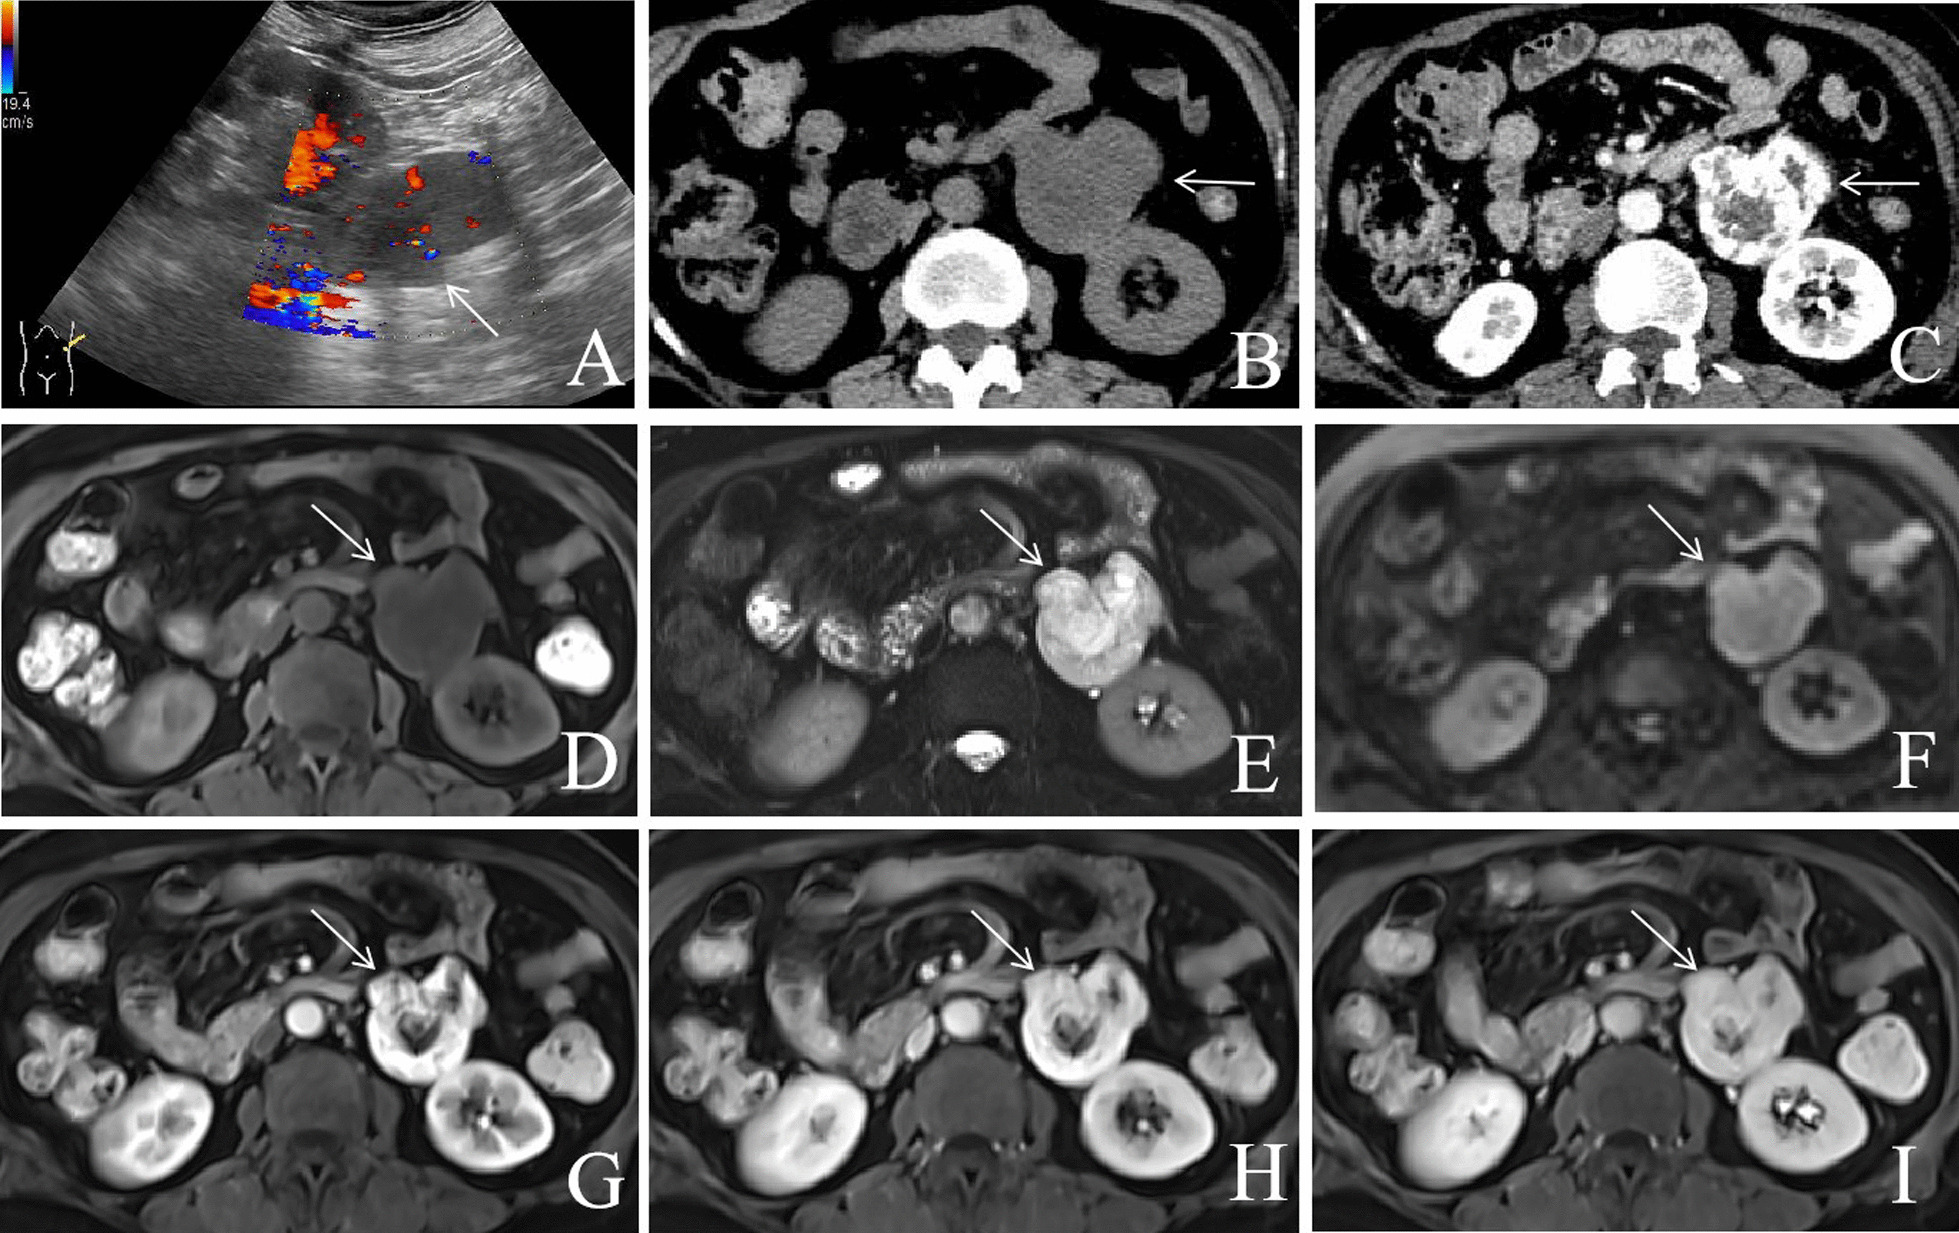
The lesion showed a hypoechoic mass with abundant blood flow signals in the perirenal space on US (A). Axial CT of the abdomen (plain scan and venous phase) showing a slightly low-density lesion (B) in the retroperitoneal space that was well-defined and with obvious enhancement (C). Axial pancreatic MRI showing a solid lesion with several cystic lesions, T1WI showed hypointensity (D), T2WI showed high-intensity (E), and DWI showed iso-intensity (F). The lesion had obvious progressive enhancement (G–I)
